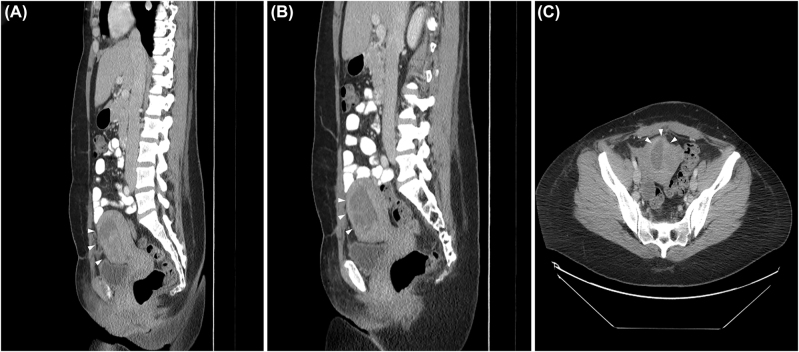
Sagittal (A, B) and axial (C) postcontrast CT images obtained on post-operative day 20. The images reveal a large heterogeneous area with patchy foci of hyperattenuation involving the anterior myometrium (white arrow heads), which corresponds to same area of abnormality on previous ultrasound and MRI imaging. These findings again support the diagnosis of large dissecting anterior intramural hematoma. The hysterotomy site is suboptimally identified on CT.
